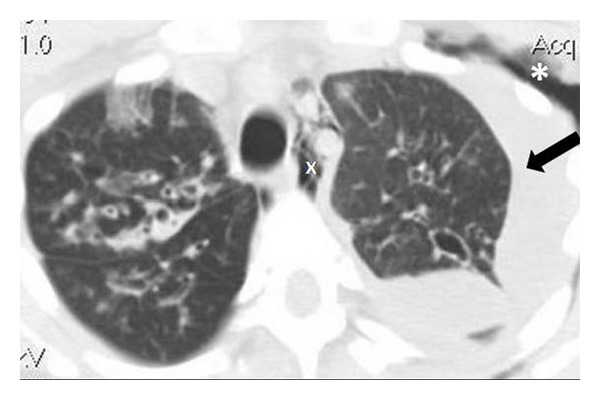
CT scan of the chest demonstrating pleural effusion (arrow), subcutaneous emphysema (asterisk), and pneumomediastinum (x). Subcutaneous air and mediastinal air are likely secondary to instrumentation.
